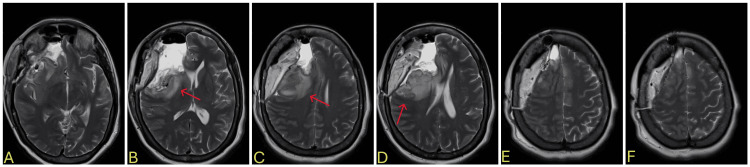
Day 2 brain MRI after the second surgery (wound exploration and resection of the recurrence) A-F: postoperative area of enhancement with overlying cerebral edema (red arrow) MRI: magnetic resonance imaging
